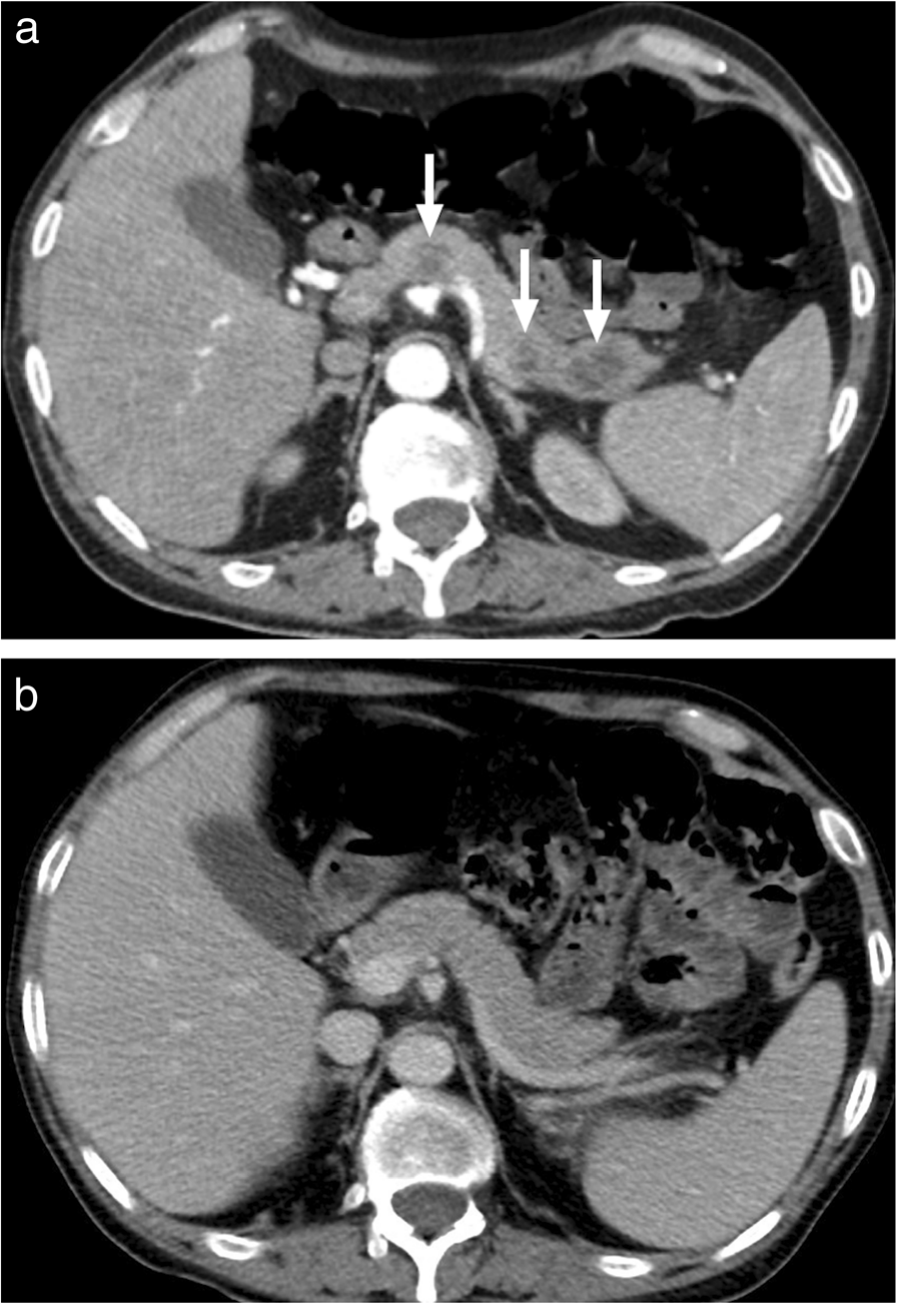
A 65-year-old male with newly diagnosed aggressive lymphoma, undergoing intense chemotherapy in intensive care unit, recently started to complain of fever and epigastric pain. Blood tests were positive for leukocytosis and mildly elevated amylase. a Axial plane postcontrast abdominal CT image demonstrates several ill-defined, hypodense lesions within the pancreatic parenchyma (arrows). There was no pancreatic duct dilation or any peripancreatic inflammation/collection. Chest CT study showed innumerable foci of septic emboli within the lung parenchyma. Pancreatic septic emboli or lymphomatous involvement of the pancreas were considered in differential diagnosis. b Follow-up abdominal CT 7 days after IV antibiotic treatment, surprisingly demonstrated almost complete disappearance of the focal pancreatic lesions
